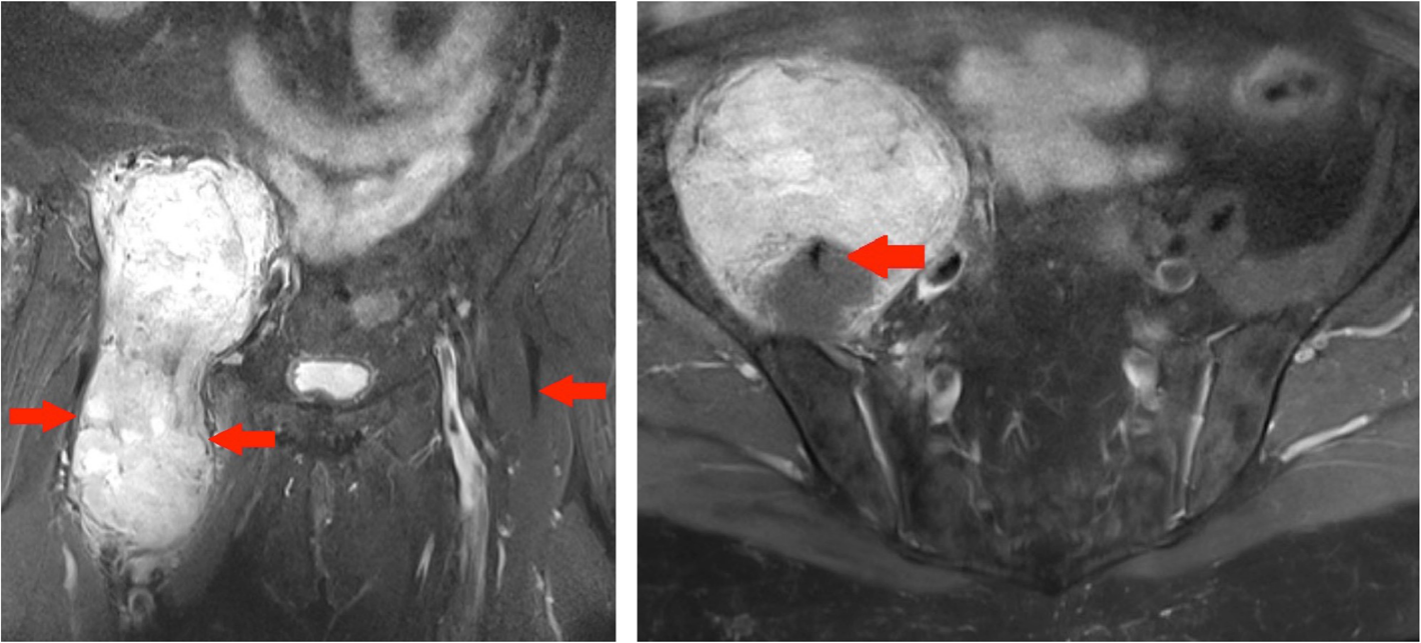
Left panel: Coronal T1 TIRM MR image of the pelvis: right-sided large heterogeneous tumour with areas of internal fat component demonstrating suppression on this fat saturated sequence. The paucity of fat components and the nodularity of the lesion suggested that radiologically this is a high grade, undifferentiated sarcoma. Right panel: Axial T1 TIRM MR image of the pelvis: the lesion is in continuity with the right iliopsoas and the red arrow outlines the iliopsoas tendon
